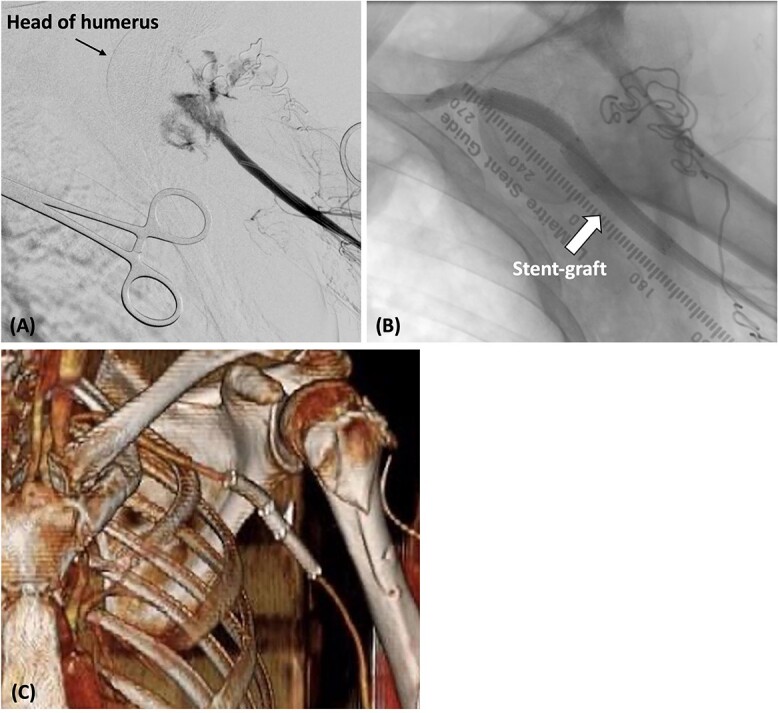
(A) The catheter was inserted from the left brachial artery, and angiography revealed the extravasation of the axillary artery. (B) Stent grafts were placed at the site of the axillary artery rupture to confirm that there was no leakage. (C) Postoperative contrast-enhanced CT revealed that blood flow in the axillary artery was maintained.
